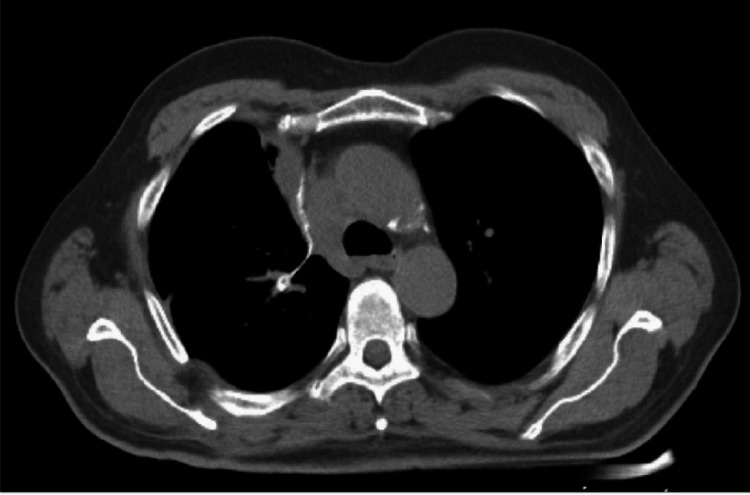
Postoperative CT image: no gross residual tumor was identified in the lung.
